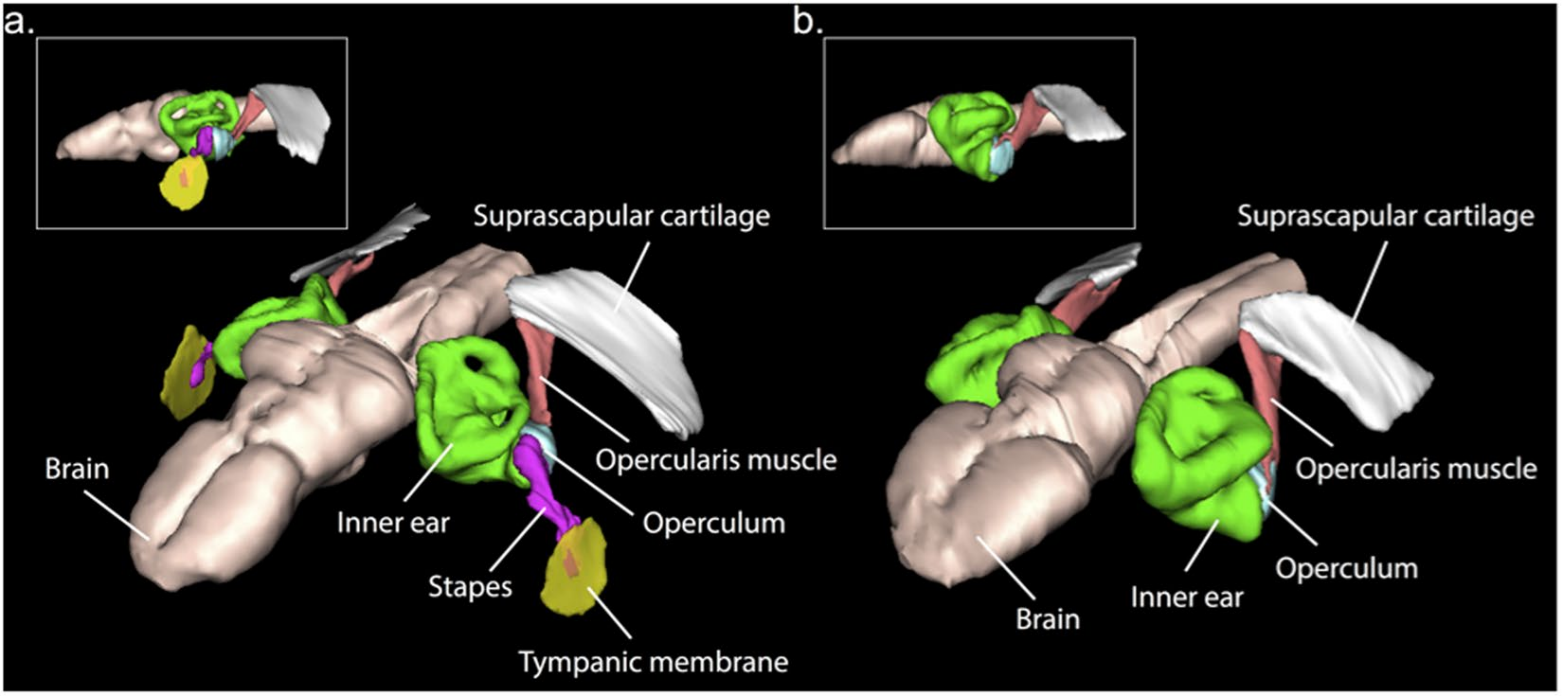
Middle ear structures in “eared” and “earless” frogs. Three-dimensional reconstructions (anterolateral view), made from micro-computed tomography data, of the middle ear structures and brains of (a) the “eared” frog *Ischnocnema parva*, and (b) the “earless” pumpkin toadlet *Brachycephalus ephippium*. Lateral views are presented in upper panels.
